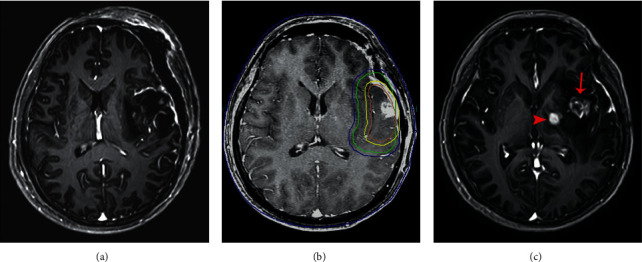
Example of NERF. A 25-year-old female with pathologically confirmed glioblastoma in the left temporal lobe. Her OS was 550 days. Axial contrast-enhanced T1-weighted image 48 h after resection (a). Axial contrast-enhanced T1-weighted image with treatment-planning overlay shows the volumes treated with 60 Gy (yellow outline) and 50 Gy (green outline) (b). The enhancing lesion (arrow) within radiation field and a NERF lesion (arrowhead) outside radiation field in follow-up MRI were detected 24 months after completion of radiotherapy (c). NERF, new enhancement beyond the radiation field.
